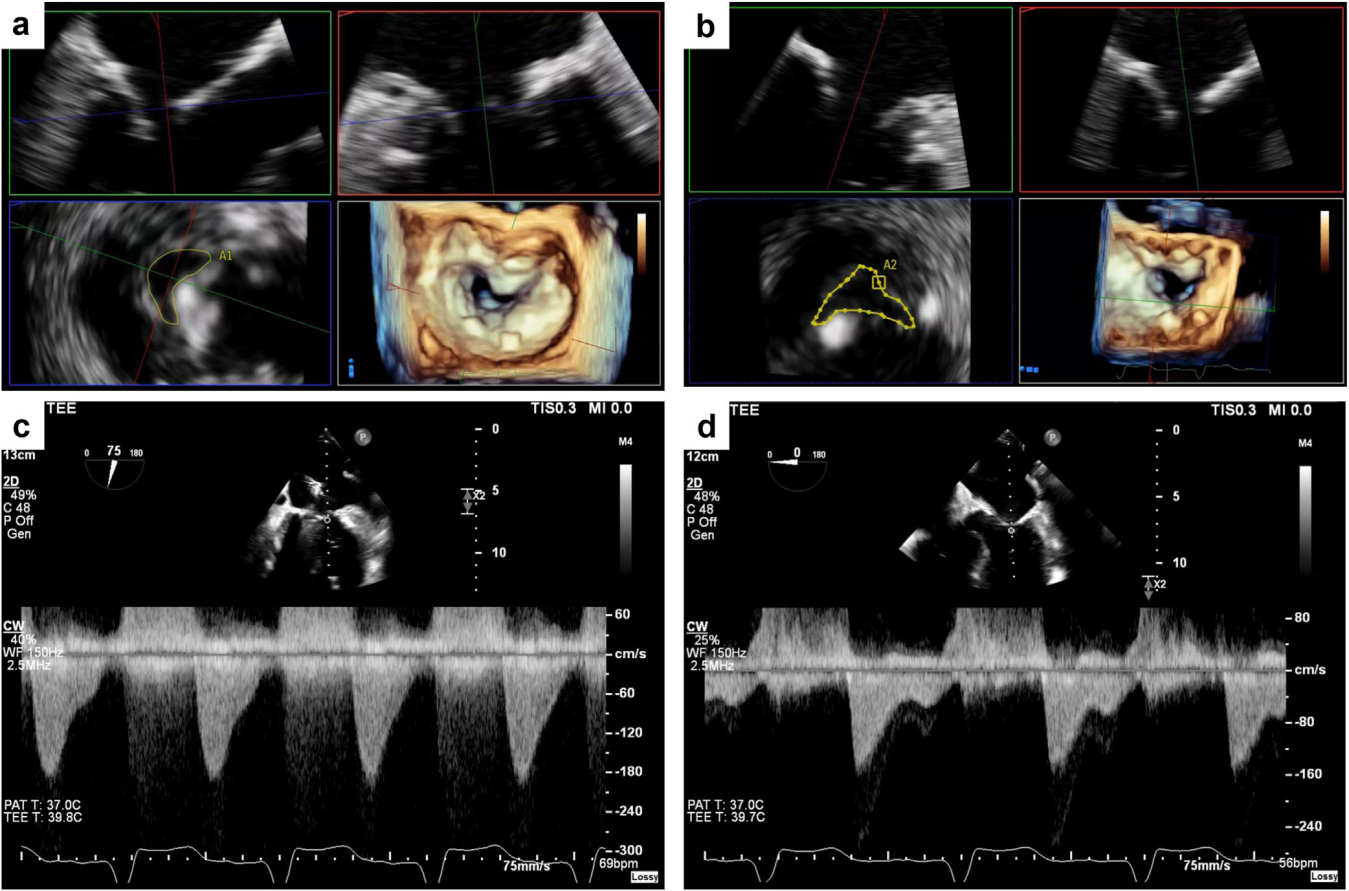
TEE measurements pre- and post-MATRIX procedure. (a) Pre-MATRIX procedure with MV area of 1.05 cm^2^ and circumference of 4.9 cm. (b) Post-MATRIX procedure with MV area of 1.65 cm^2^ and circumference of 6.8 cm. (c) Pre-MATRIX procedure with elevated peak/mean MV gradient of 16/7 mmHg. (d) Post-MATRIX procedure with reduced peak/mean MV gradients of 8/2 mmHg. Abbreviations: MATRIX, Mitral Annulo-TRIpsy in eXtreme risk patients; MV, mitral valve; TEE, transesophageal echocardiogram.
